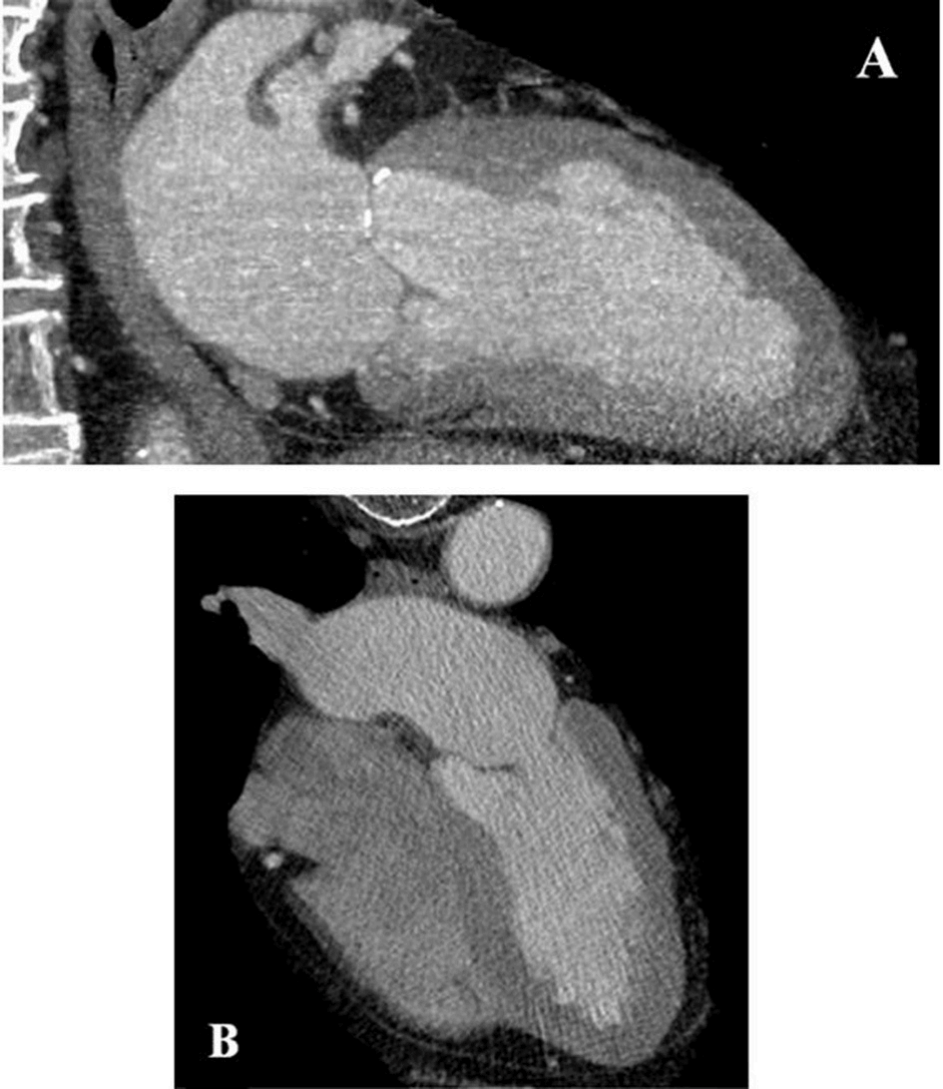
Cardiac computed tomography of the left ventricle vertical view (A) and four chamber view (B) without any evidence for a left ventricular thrombus after 4 weeks.
